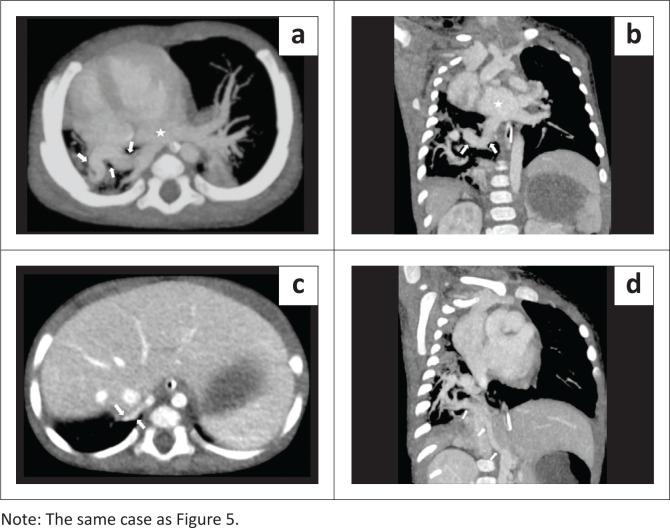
(a) Axial maximum intensity projection (MIP) image and (b) Coronal MIP image of the contrast enhanced computed tomography (CECT) chest reveal an anomalous unilateral single right pulmonary vein (arrows) that is tortuous and draining into the left atrium (white star). (c) Axial CT image and (d) oblique coronal MIP image of the CECT chest show an aberrant systemic artery (arrows) arising from the abdominal aorta and supplying the right basal lung parenchyma.
